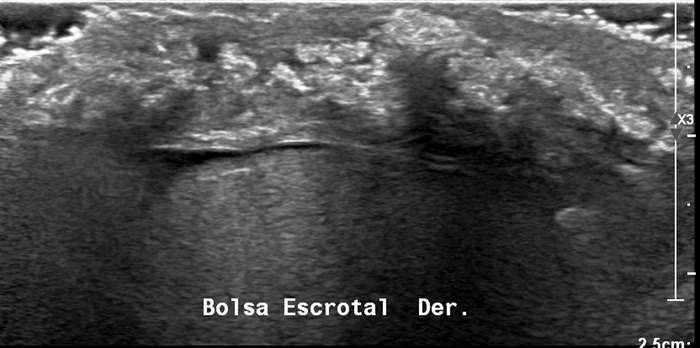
Ultrasound shows an increase in scrotal thickness; areas with increased echogenicity correspond to calcified nodules, some of which project posterior acoustic shadow corresponding to a gouty tophus.
